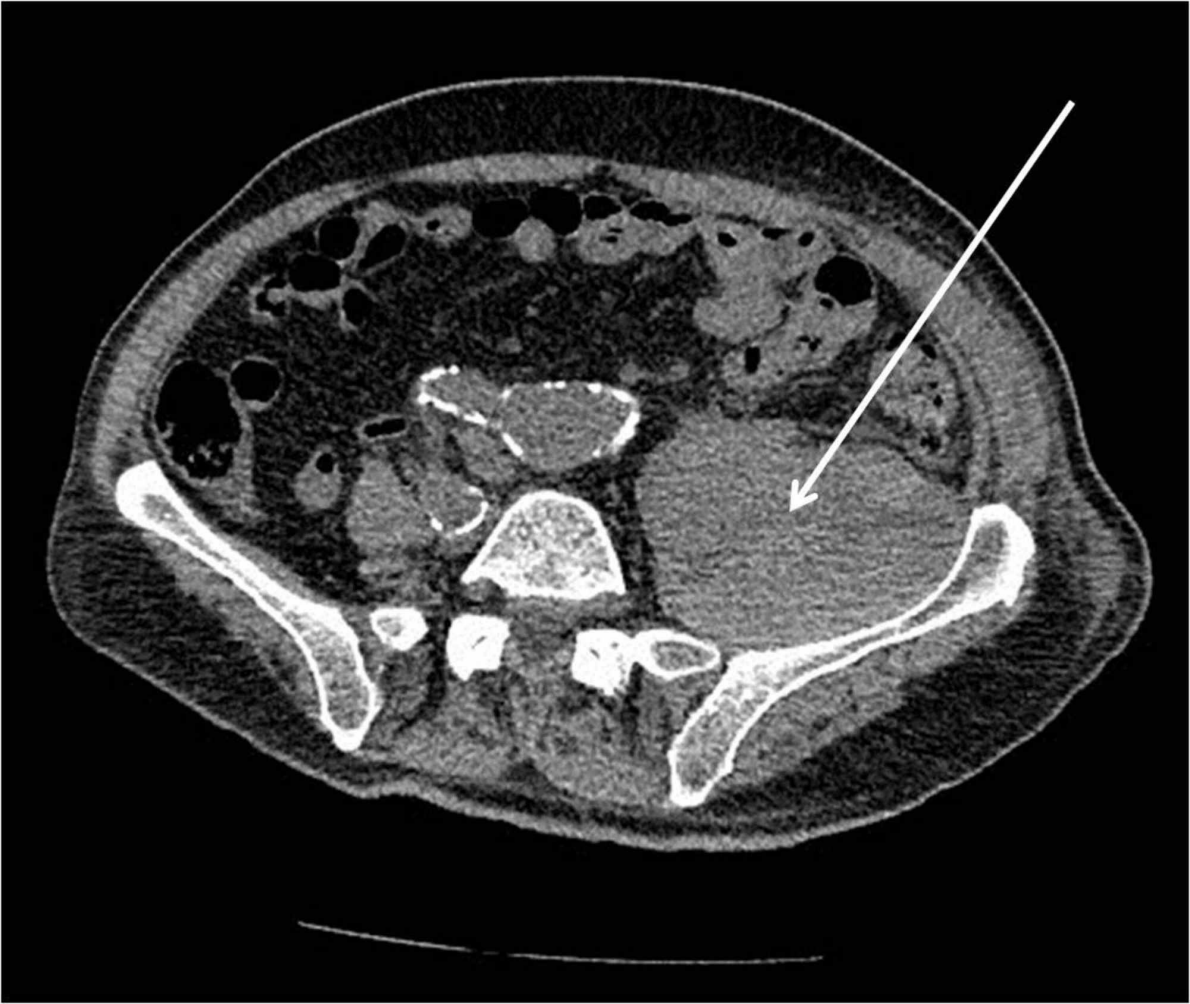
CT scan revealing a left iliopsoas haematoma (arrow) on day of admission.
